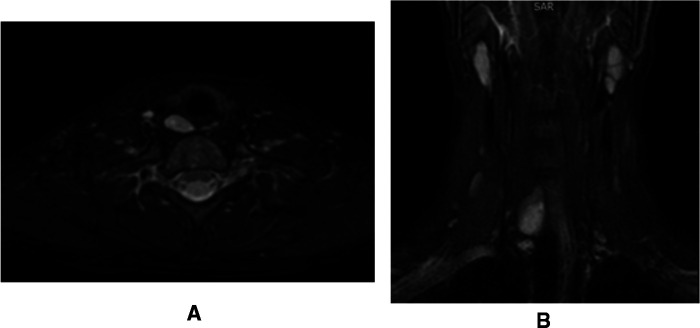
Parathyroid adenoma visualization on magnetic resonance imaging. (A) Transversal projection; (B) Coronal projection.
